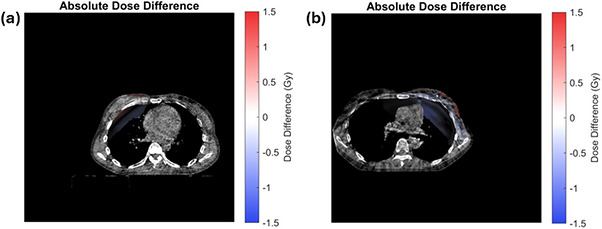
Representative axial absolute dose difference maps (Δ = deformed pCT − CBCT) for (a) Halcyon CBCT and (b) TrueBeam CBCT.
